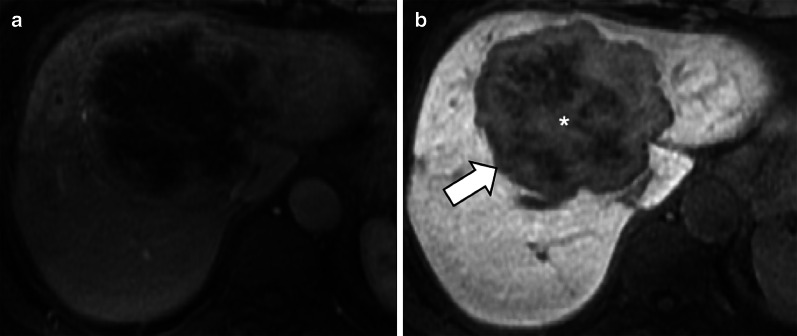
A 71-year-old woman with cholangiocarcinoma. Gadoxetate disodium-enhanced MRI shows an intrahepatic mass-forming cholangiocarcinoma with (a) continuous rim enhancement on hepatic arterial phase and (b) a target pattern on hepatobiliary phase with peripheral hypointense rim (arrow) and inhomogeneous contrast media uptake with a central enhancing area (asterisk) likely related to fibrous stroma
